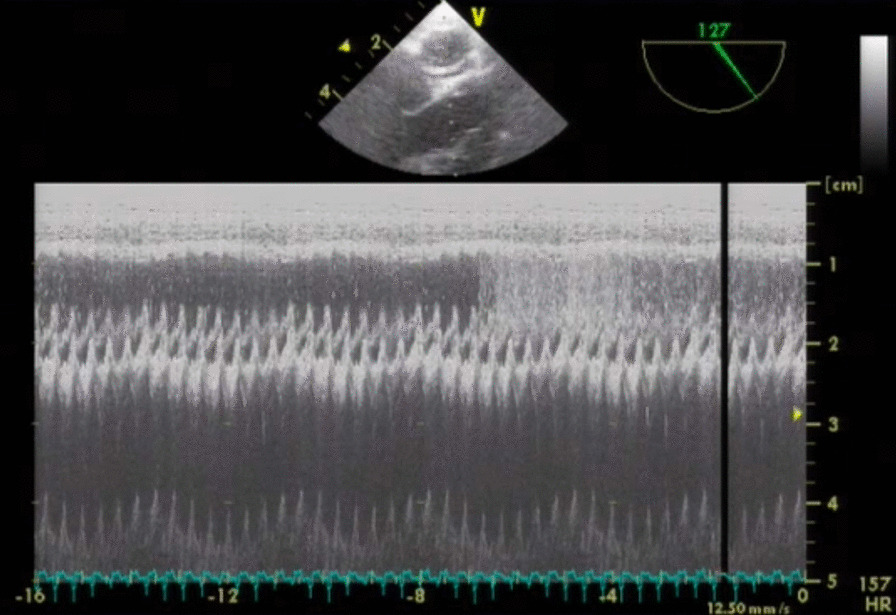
Transesophageal M-mode echocardiography through the pulmonary artery and the left ventricular outlet tract. Transesophageal M-mode echocardiography (TEE) visualizing the pulmonary artery (above) and the left ventricular outlet tract (below) during intraosseous fluid infusion. We used continuous TEE to help confirm intraosseous placement and to monitor for intracardiac shunt. Horizontal sweep rate was set to 12.50 mm/s
